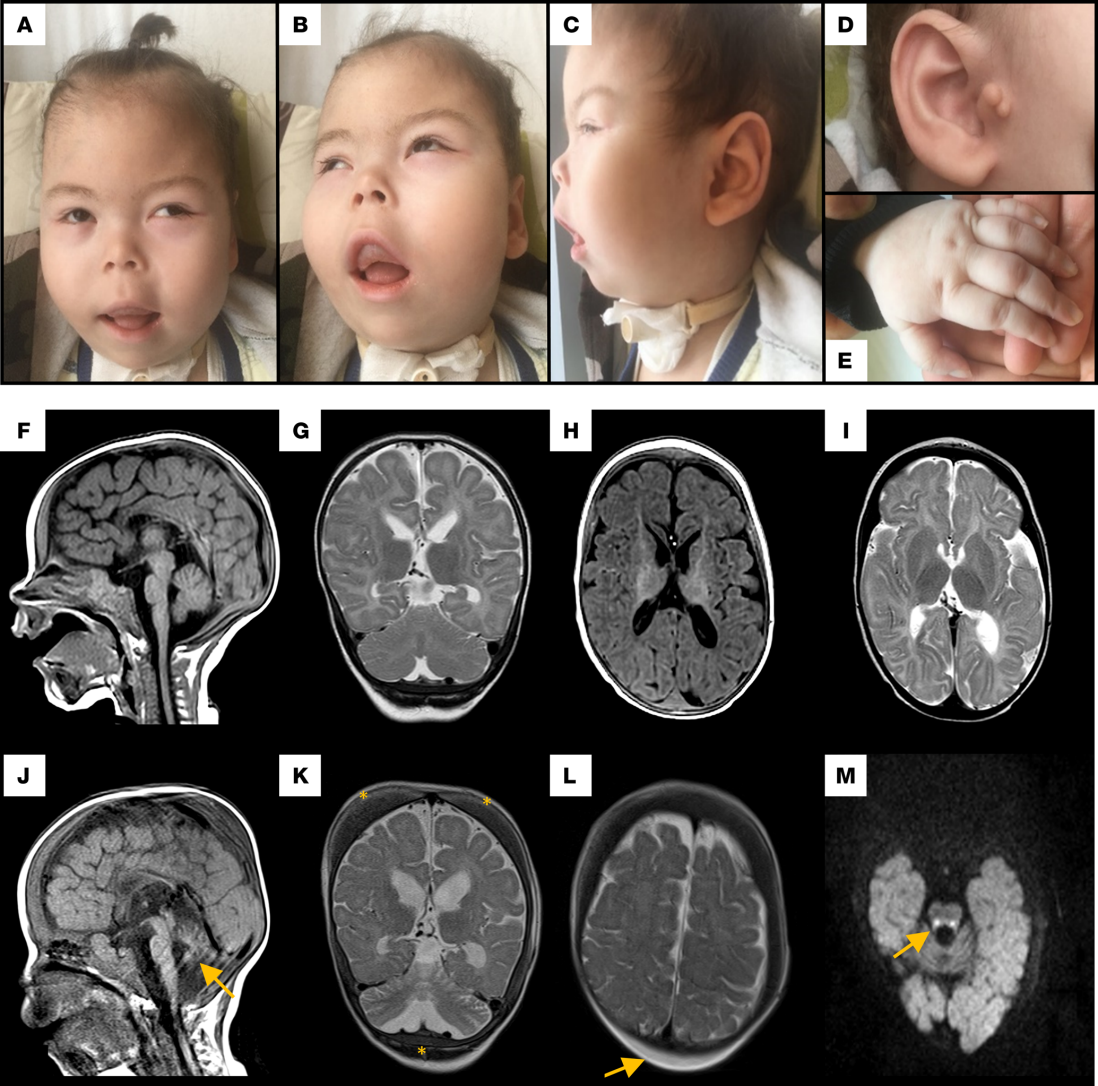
Clinical findings and MRI results of individual V-8. (A–C) Note full cheeks, small nose with hypoplastic alae, hypoplastic malar region, long philtrum, arched and thin upper lip, gingival hypertrophy, right preauricular tags (D), and tapering fingers (E). (F–I) Cranial MRI at the age of 7 months and 14 months (J–M). T1-weighted sagittal (F and J) and T2-weighted coronal images (G and K) showing thin corpus callosum, progressive cerebellar atrophy (yellow arrow), cerebral atrophy with enlarged lateral ventricles, and enlargement of calvarial bones demonstrated by increased bone marrow intensity (yellow asterisks). T1- and T2-weighted transverse images with diffuse hypomyelination pattern (H and I). T2-weighted transverse image shows lack of myelination and increased bilateral parietal subcutaneous fat (yellow arrow) (L). Transverse diffusion weighted image b = 1,000 (M) demonstrates restricted diffusion in dorsal brainstem pathways at the level of the superior cerebellar peduncle (yellow arrow).
